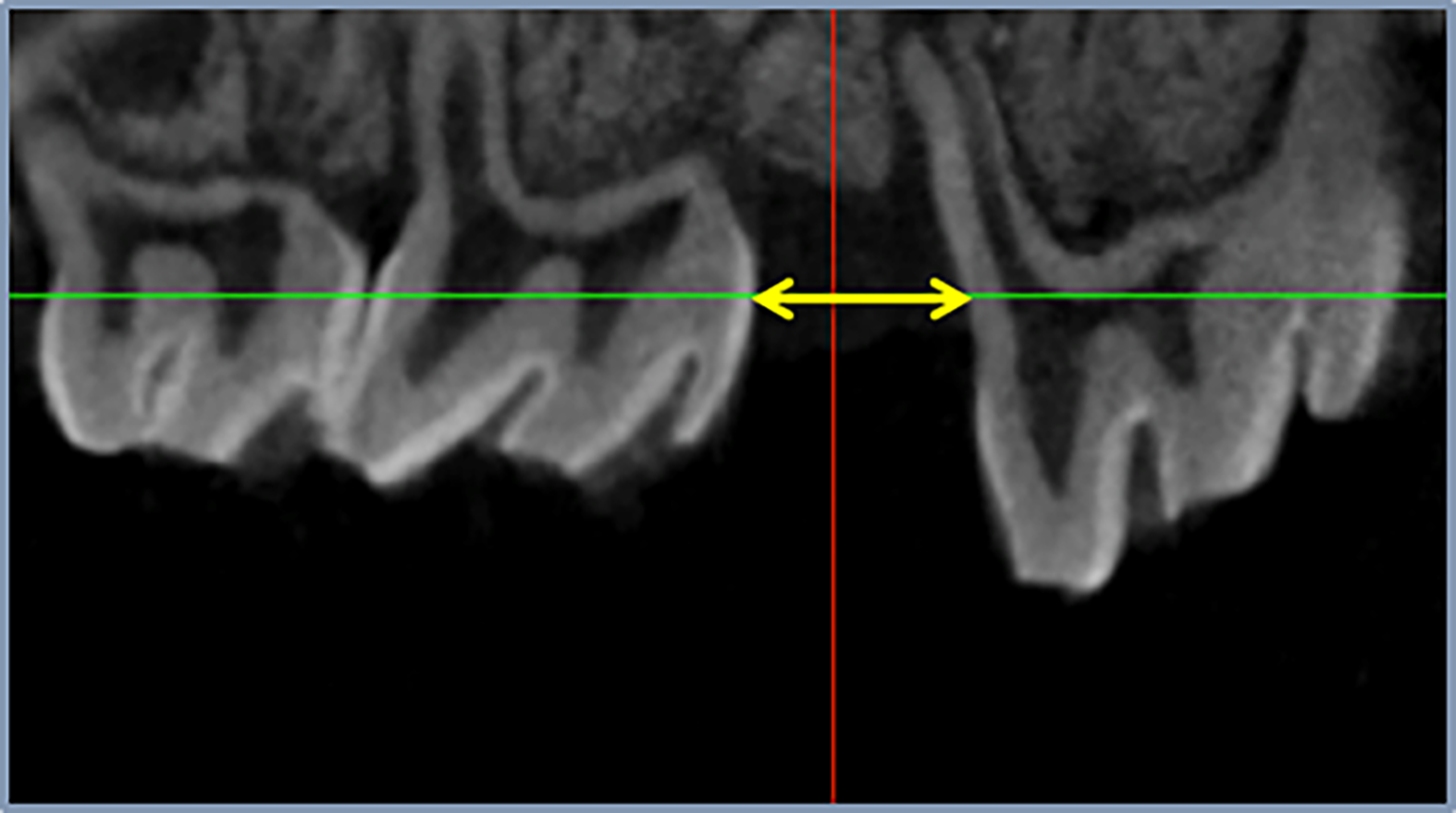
Observation of tooth movement. After 30 days, the amount of tooth movement was measured by means of computed tomography.
